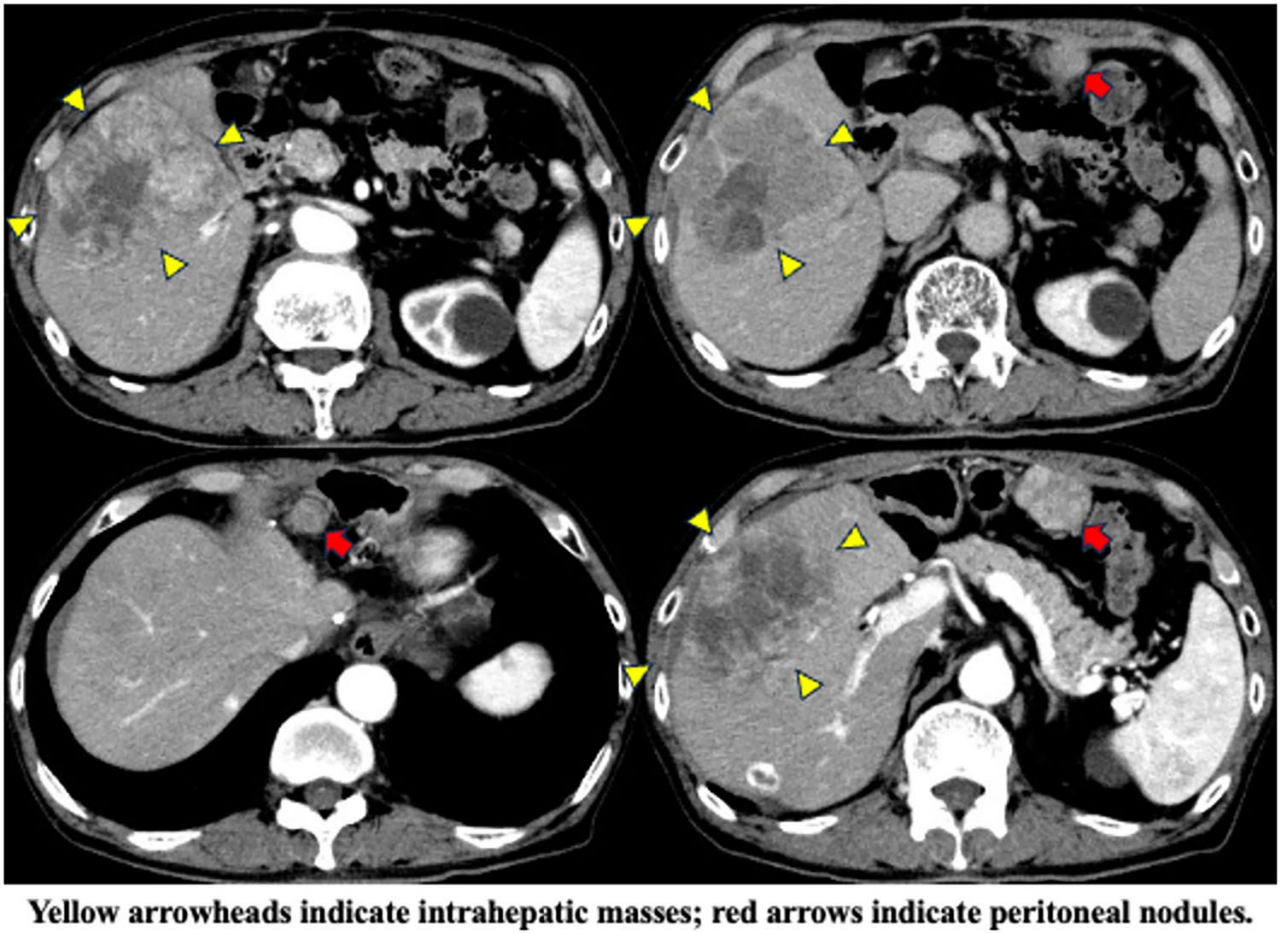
Contrast-enhanced computed tomography upon admission to our hospital
